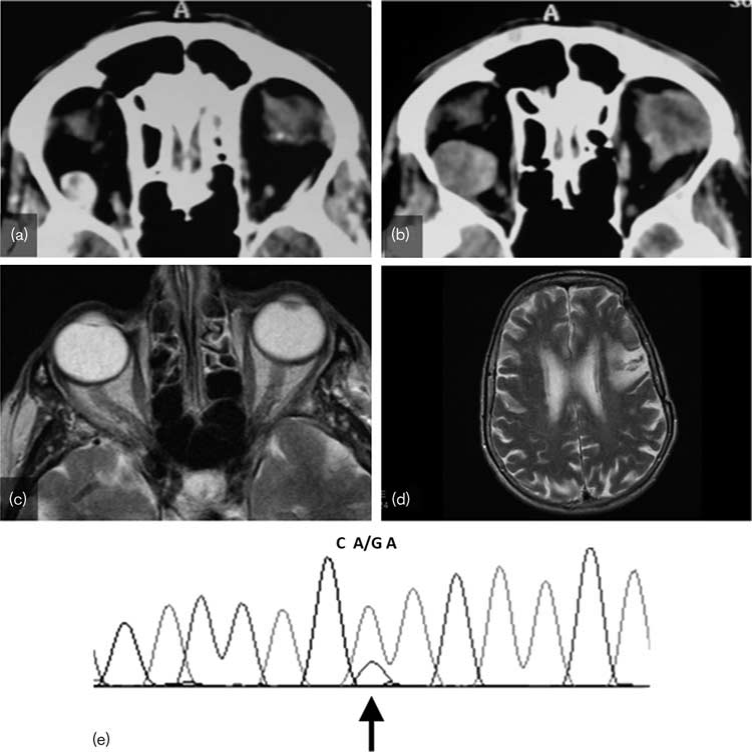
Imaging of orbital and intracranial disease in a patient with disseminated cutaneous malignant melanoma. (a, b) Orbital computed tomography at presentation showed a well-defined soft-tissue mass supero-temporally in the right retrobulbar fat. (c) MRI showed no recurrence of orbital disease 14 years after excision of the orbital mass and radiotherapy. (d) MRI showing gliosis at the site of previous surgical excision and radiotherapy for left frontal metastases, but without disease recurrence at 15 years after treatment of the intracranial disease. (e) Sequencing of DNA extracted from the patient’s metastatic melanoma excised from her orbit, this showing a heterozygous point mutation (c.A182G; p.Q61R) (arrowed) in exon 3 of *NRAS*.
